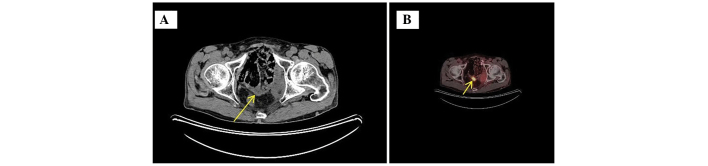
(A) Axial reconstruction showing CT imaging (the arrow indicates pelvic lymphadenopathy). (B) Axial ^18^F-fluorodeoxyglucose-positron emission tomography/CT scan showing the maximum standardised uptake value: 4.2 (the arrow indicates pelvic lymph node metastasis). CT, computed tomography.
